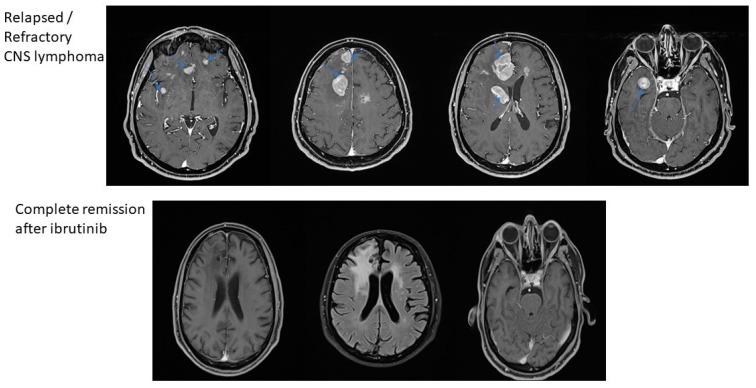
MRI-based remission of CNS lymphomas treated with ibrutinib.
